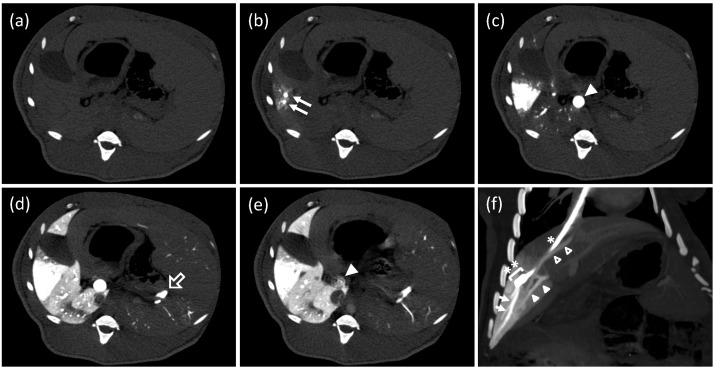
Computed tomography (CT) study during a hydrodynamic injection from a catheter, which was placed at a branch of the hepatic vein. (a–e) CT images at the same axial level were repeatedly obtained with a 0.5 s interval before, during, and after a regional hydrodynamic injection of contrast medium at a constant speed of 20 mL/s in 7.5 s. Each image was taken before the injection, (a); 1 s, (b); 2.5 s, (c); 7.5 s, (d); and 12 s, (e); after the injection. Closed arrows: hepatic vein (HV) branches in the target area; closed arrowhead: main trunk of the portal vein (PV); open arrow: splenic vein. (f) An oblique multi-planar reconstruction image immediately after the end of a hydrodynamic injection before removing a balloon occlusion. The coronal image was reconstituted with a 17.7-mm thickness. A catheter (*) with a balloon () was placed in the branch of hepatic vein via the right jugular vein. Arrows: periphery of the target HV; closed arrowheads: portal vein of the target area; open arrowheads: another hepatic vein beginning from the inside of the target area.
